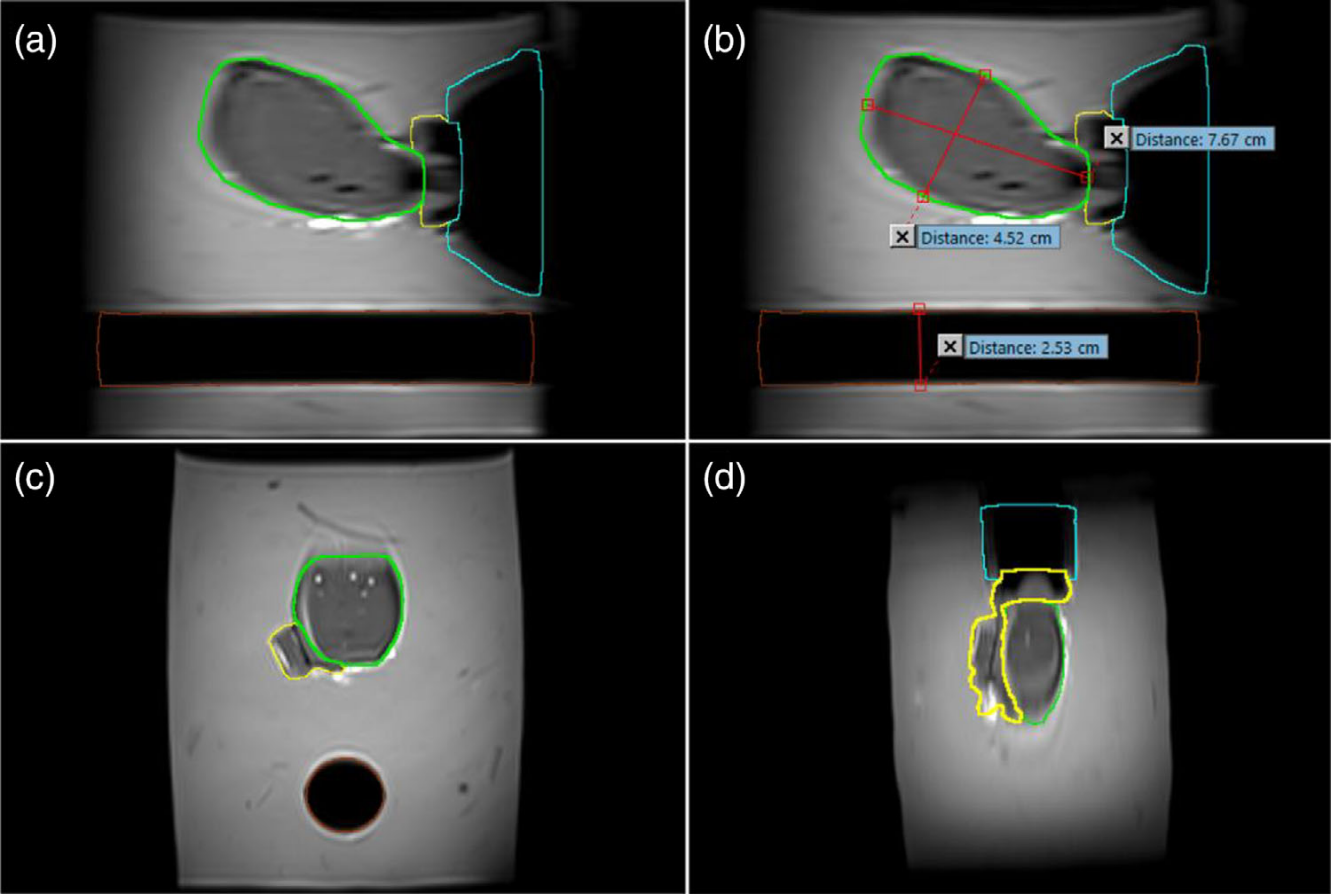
(a) Pre‐implant sagittal T2w‐MR image showing uterus (green), cervix + extracervical CTV_HR_ (yellow), vaginal canal (cyan), and rectum (red); (b) Measurements of uterus and rectum on BrachyVision TPS; (c) transverse T2w MR; (d) coronal T2w‐MR.
